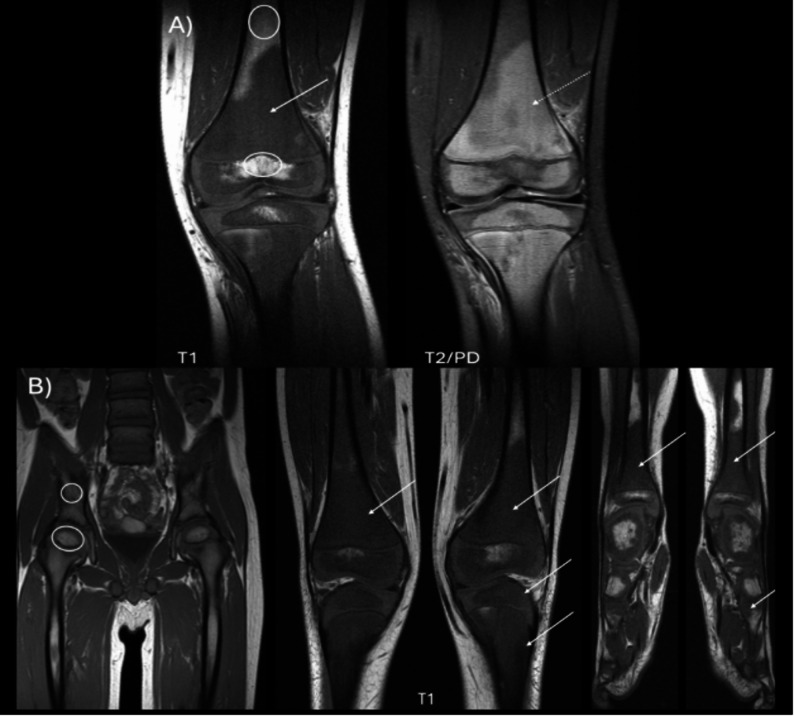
(A) In patient 5, MRI of the left knee shows pathologically low T1w signal (arrow) and high T2w signal intensity in the bone marrow (dotted arrow), compared with normal marrow (circles). (B) In the same patient, MR images from the second examination, performed eight days later, show normal pelvic bone marrow (circles), whereas the bone marrow of both knees and distal lower legs demonstrates confluent lesions with complete loss of fatty marrow (arrows). This case is representative of the diagnostic challenge because an initial iliac bone marrow puncture showed no evidence of leukemia; MRI-guided biopsy from the left tibia subsequently confirmed ALL
